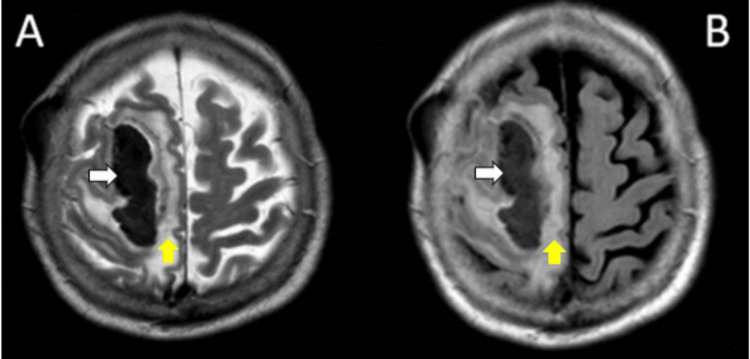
Axial T2W (A) and FLAIR (B) MRI sequences of the brain showing a hypointense signal (white arrows) on the right frontal lobe with perilesional vasogenic edema (yellow arrows). T2W: T2-weighted image; FLAIR: fluid attenuated inversion recovery; MRI: magnetic resonance imaging
